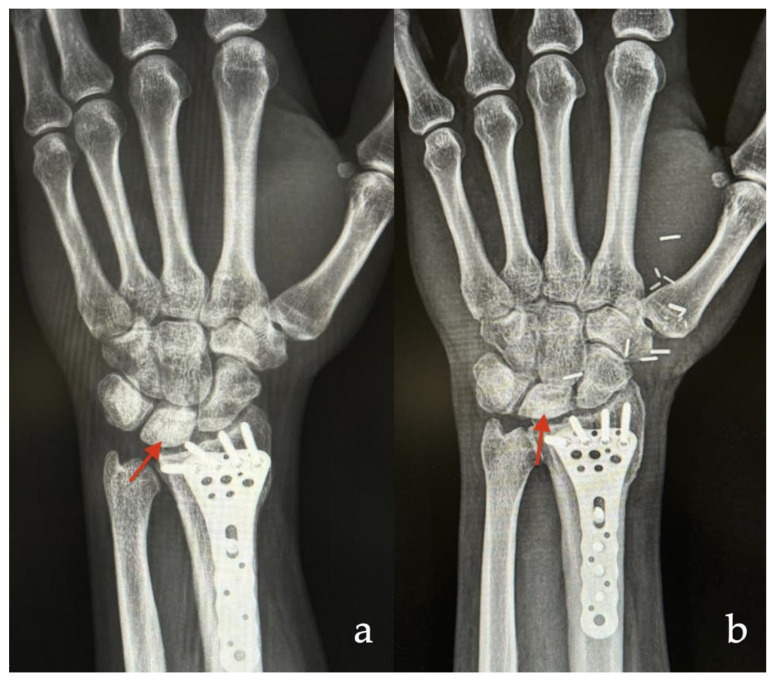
Male patient who underwent vascularized bone reconstruction due to Kienböck’s disease on the left hand. (a) Preoperative X-ray—recurrence of partial avascular necrosis of the Lunate (red arrow) two years after radial shortening. (b) Postoperative X-ray—one year after carpal bone reconstruction with free MFC bone flap (red arrow) assisted with 3D technology.
